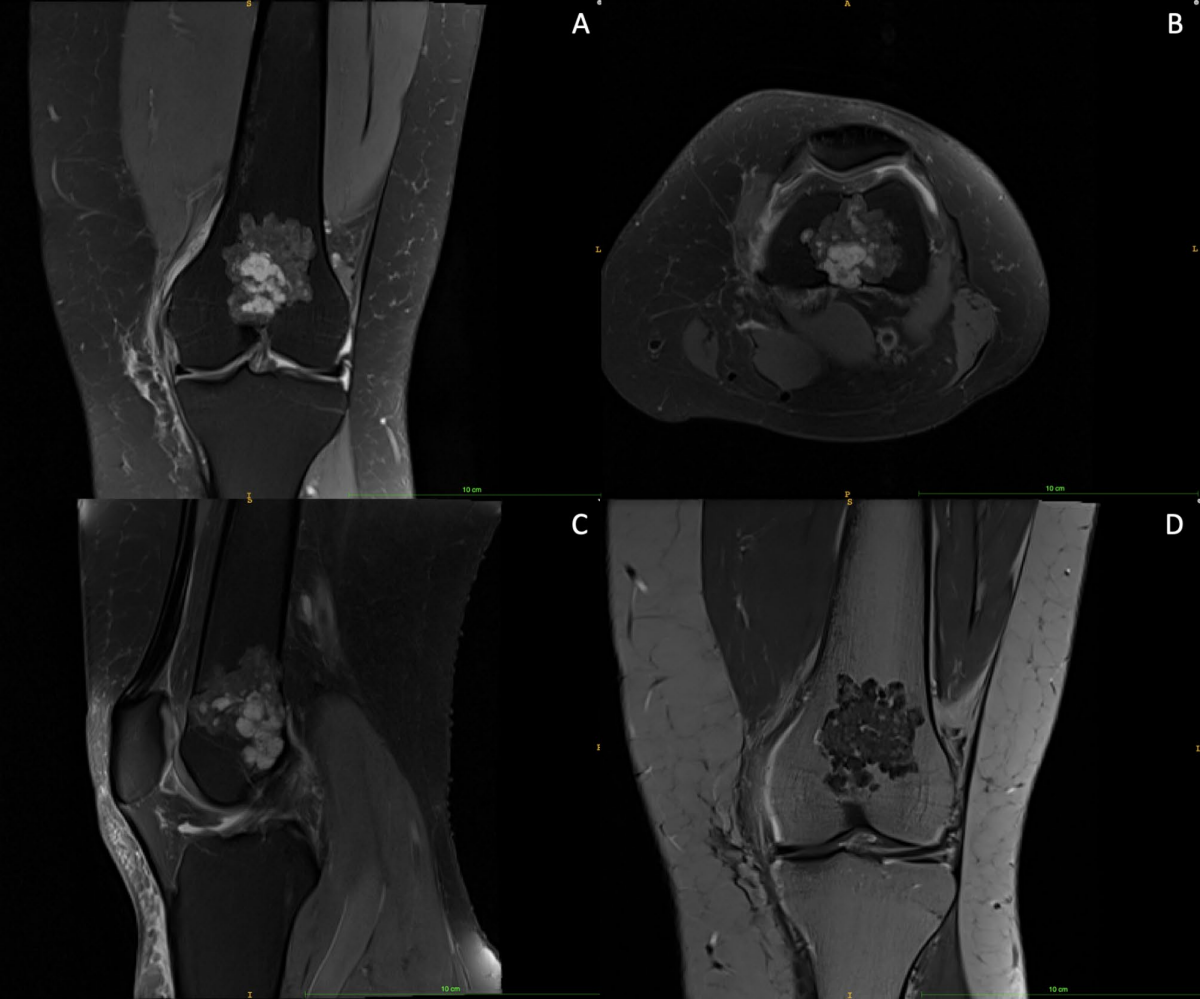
ACT of the left femur: (A) proton density, fat suppression, coronal, turbo spin echo; (B) proton density, fat suppression, transversal, turbo spin echo; (C) proton density, fat suppression, sagittal, turbo spin echo; (D) t1, coronal, turbo spin echo (year of MRI: 2016)
